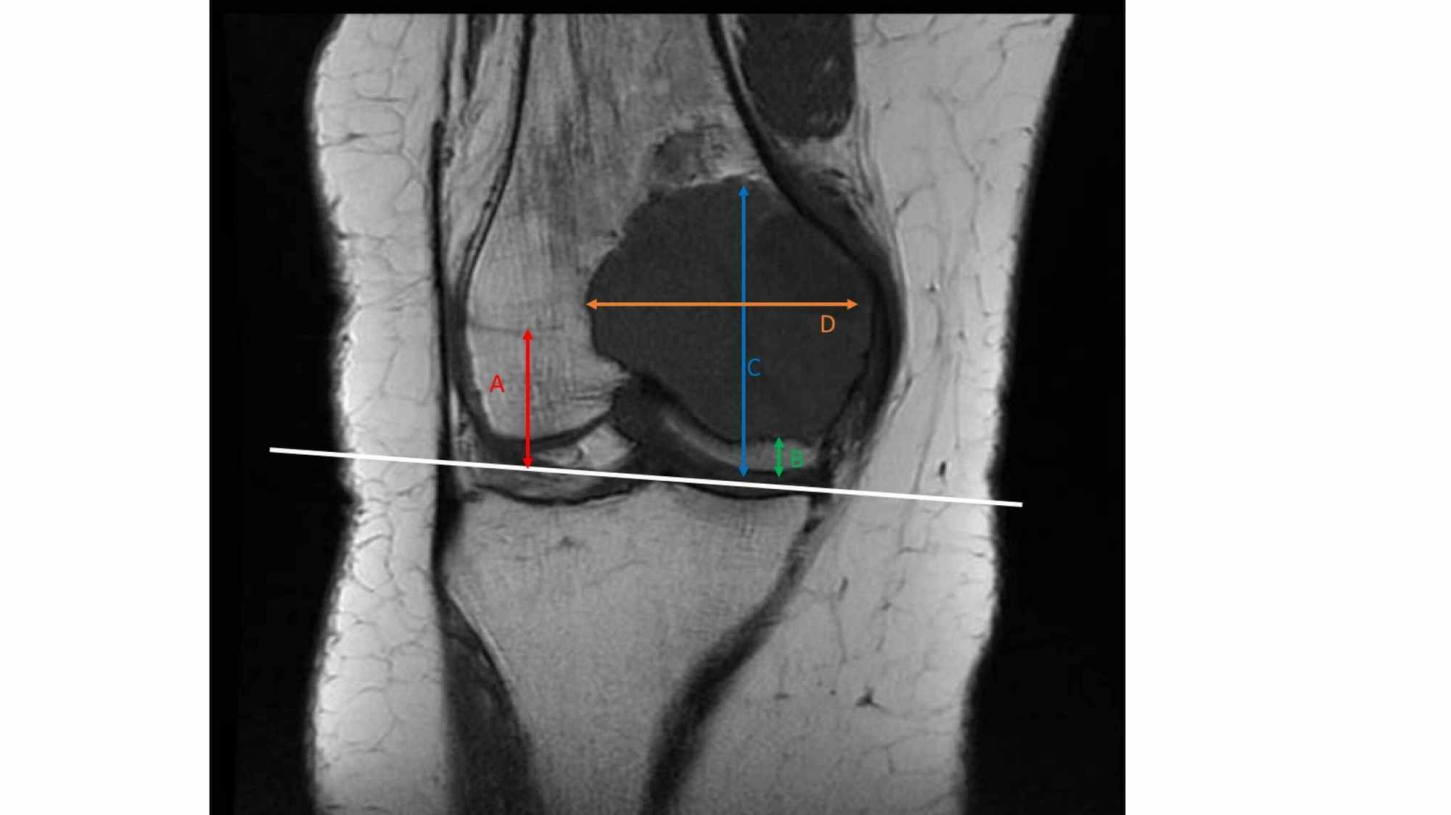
Radiological measurements Coronal T1 MRI scan showing the measurements taken. From the joint line of the affected part of the bone, the distance to the physeal scar (A), inferior margin of the tumour (B) and superior margin (C) of the tumour were measured. The width of the tumour (D) was also measured. All measurements were in pixels. MRI: magnetic resonance imaging
